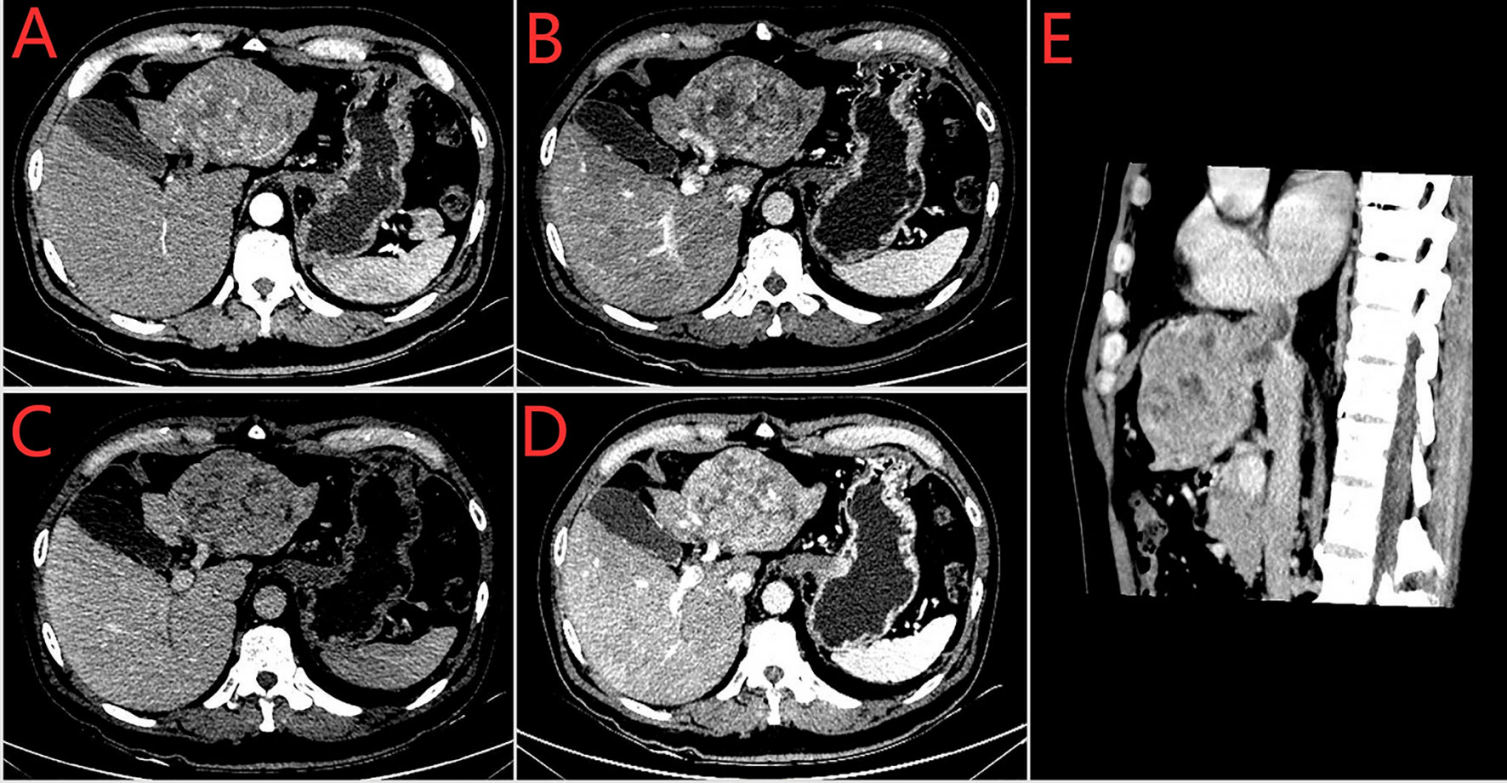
Preoperative enhances-CT of the abdomen (2016–7–7). (A) arterial phase (B) venous phase (C) delayed phase (D) The left branch of portal vein was invaded (E) Sagital plane; Tumor thrombus of vena cava.
